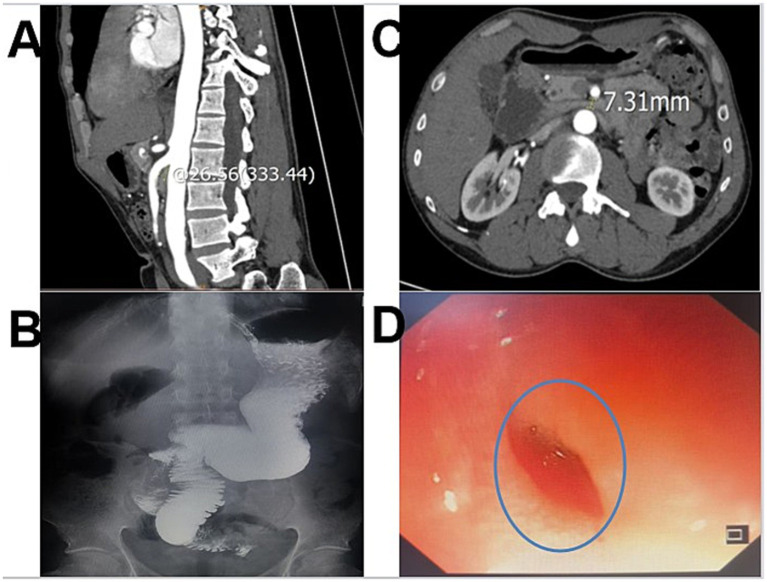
(A) Sagittal CT image demonstrating compression of the duodenum, consistent with SMAS. (B) Upper gastrointestinal barium study revealing duodenal obstruction. (C) Traverse CT image showing duodenal compression, further supporting the diagnosis of SMAS. (D) Gastroscopy revealed a duodenal ulcer with concomitant stenosis.
